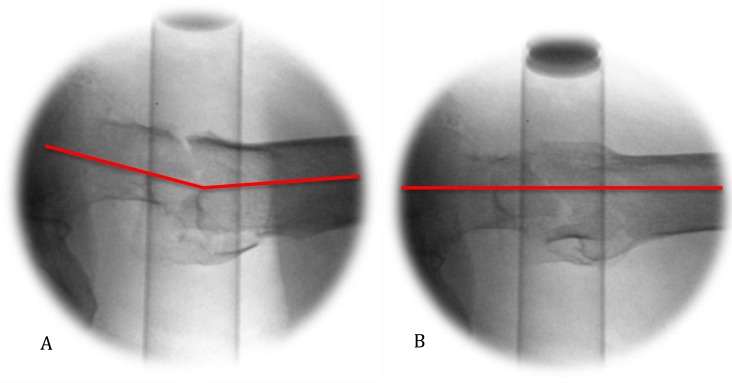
Lateral radiographs of the right femoral neck taken at (A) neutral and (B) 20 degree angulation of beam.
